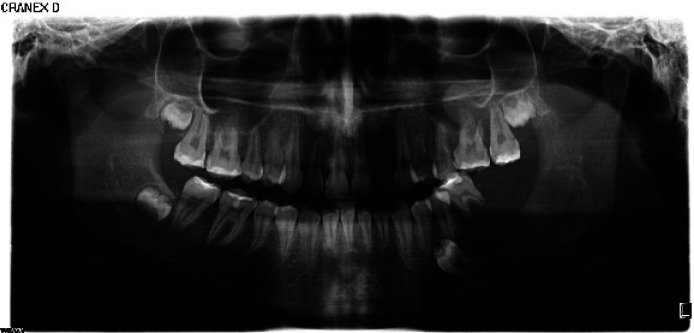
The panoramic radiograph showing a well-defined radiolucent lesion in the left mandibular body
